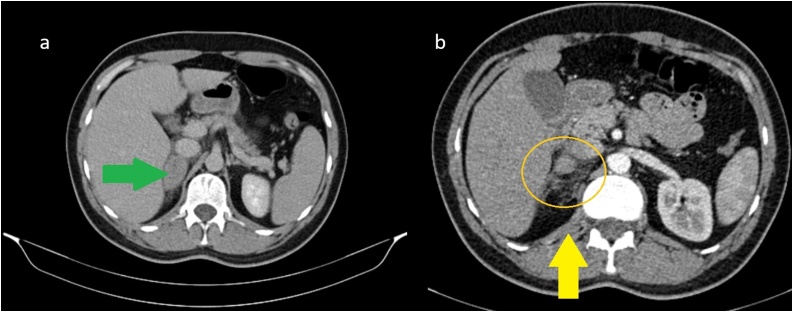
Oval, well-defined lesion (4.2 × 2.7) with high density (green arrow) and crus thickening (yellow arrow), indicative of acute AH in the initial abdominal CT. No contrast extravasation is noticed. Periadrenal fat stranding appears inferiorly (circle).
